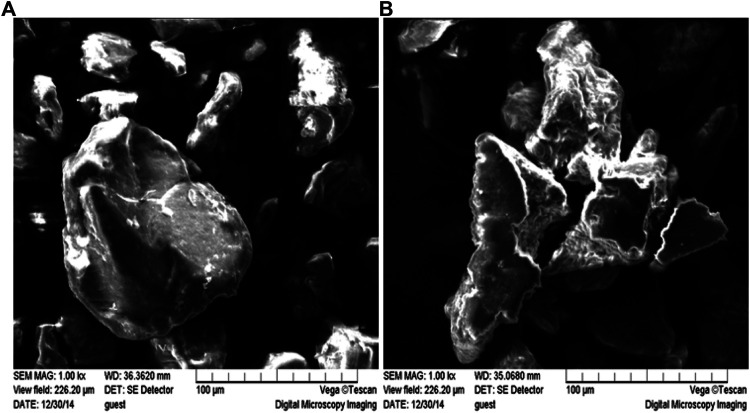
Effects of ultrasound pretreatment of different durations on the microstructure of XAC. (A) Original (0min) non-sonicated XAC; (B) Ultrasound pretreated XAC for 40 min (50 kHz, 0.40 W/cm^2^).
